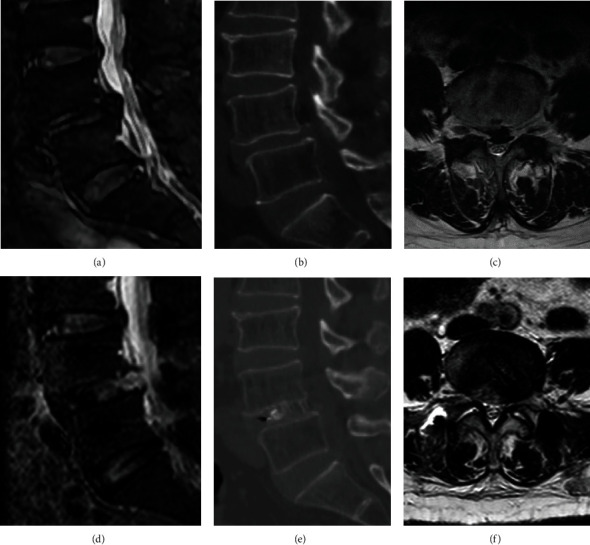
Case examples with pre- and postoperative magnetic resonance imaging (MRI) scans and computed tomography (CT) scans. A 73-year-old female patient was diagnosed as lumbar spondylolisthesis before operation. Preoperative sagittal MRI scan (a), preoperative sagittal CT scan (b), preoperative transverse MRI scan (c), postoperative sagittal MRI scan (d), postoperative sagittal CT scan (e), and postoperative transverse MRI scan (f).
